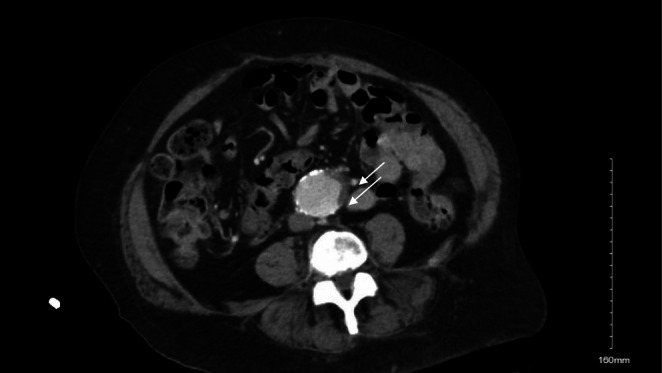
Contrast‐enhanced CT of the abdomen and pelvis demonstrating a stable 3.9 cm infrarenal abdominal aortic aneurysm (white arrow) with no acute intra‐abdominal abnormality, evidence of bowel obstruction, or organized fluid collection.
